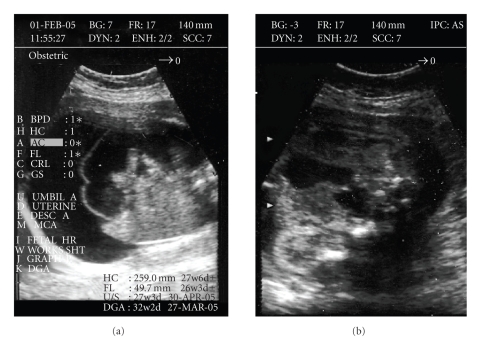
(a) Prenatal ultrasound of the fetus with significant ascites.
